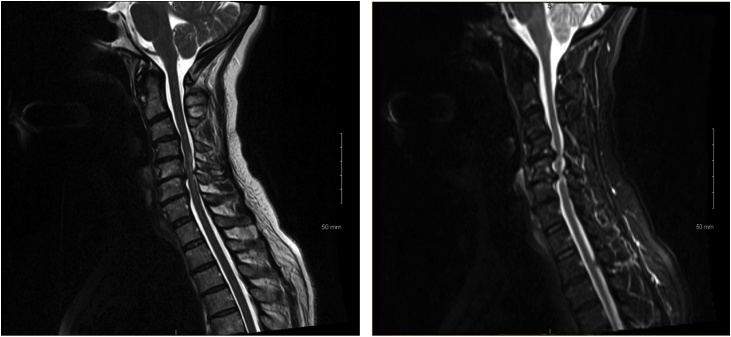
Preoperative MRI Scans showing cervical spine cord compression.
